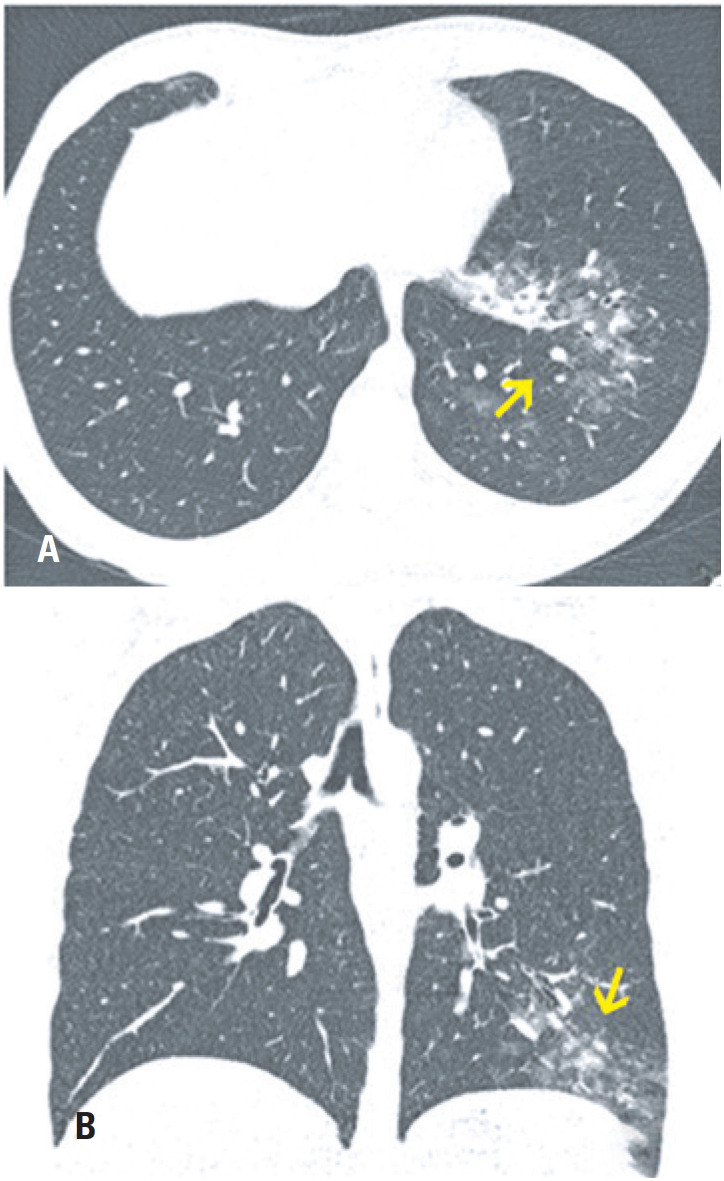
A 28-year-old male patient complaining of cough, headache and fever for 4 days. Computed tomography (A) Axial section and (B) Coronal section: thickening of bronchial walls, associated with confluent bronchocentric ground-glass opacities in the basal segments of the left lower pulmonary lobe. Such findings are compatible with a pulmonary infectious process of bronchopneumonia. Confirmed by respiratory C-reactive protein test:
